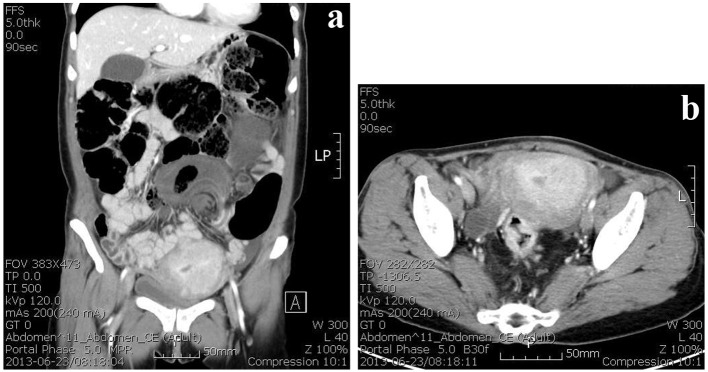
CT findings show whirl sign with rotation of inferior mesenteric vessel was identified (a). The rectum had irregular wall thickening (b).
